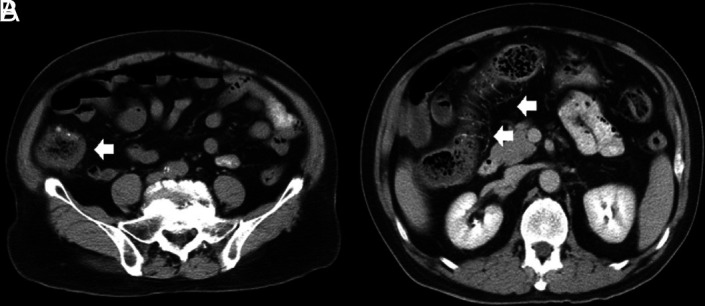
Abdominal computed tomography showing thickened colonic wall with marked nodular calcifications along the wall of the ascending colon (A, arrow) and transverse colon (B, arrows).
